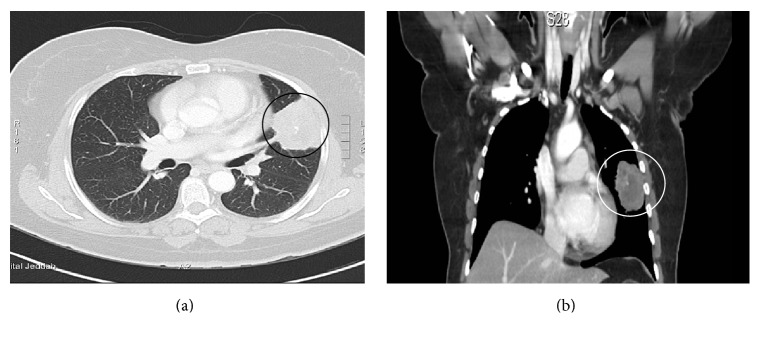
(a) Axial and (b) coronal contrast-enhanced chest CT scan showing malignant looking pleural-based lobulated left upper lobe soft tissue.
